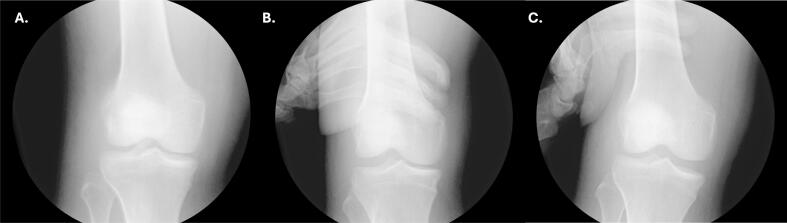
AP radiographs of the right knee during EUA without applied stress (A), applied varus stress (B), and applied valgus stress (C).
